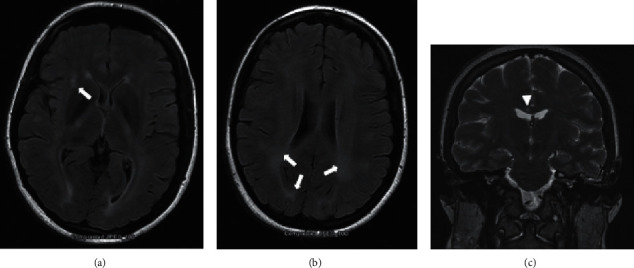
(a) and (b) Axial MRI brain without contrast demonstrating patchy and confluent FLAIR hyperintensity throughout the white matter, markedly abnormal for age (arrows). (c) Coronal MRI brain FLAIR sequence demonstrating a lesion in the corpus callosum (arrowhead), performed prior to her presentation to our facility.
